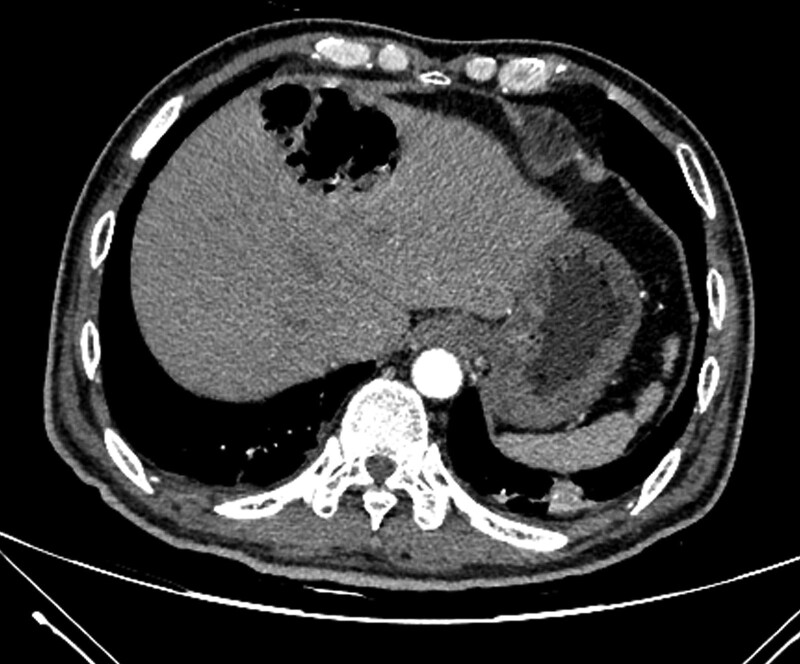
Enhanced abdominal computed tomography 2 days later showed the emphysematous alveolarization of liver parenchyma with some fluid collection around the lesion.
